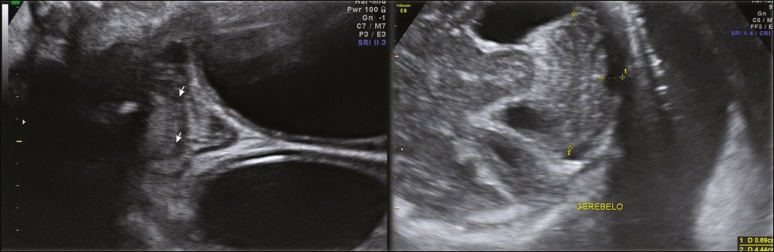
Coronal ultrasound of a fetus with rhombencephalosynapsis, showing that the foliations of the cerebellar hemispheres are continuous in the middle portion of the cerebellum, and that there is no observable vermis (arrowheads). Axial ultrasound can show a cerebellum with a transverse diameter that is normal or below the 10th percentile for gestational age and with a triangular shape.
